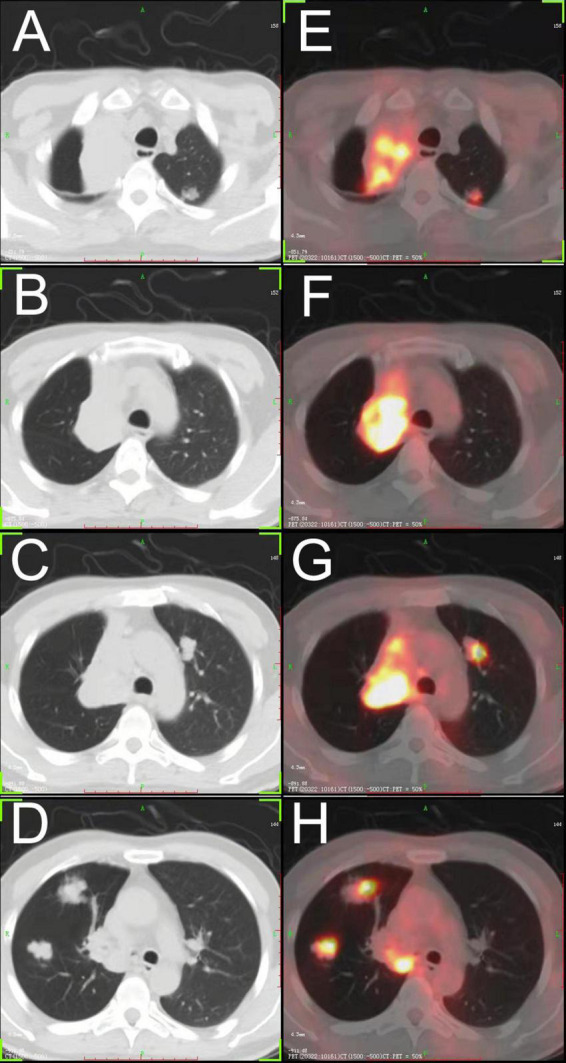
(A–D) Chest CT showing a large tumor in the hilum of the right lung, and multiple bilateral pulmonary nodules. (E–H) FDG- positron emission tomography/CT showing high accumulation of FDG in each lesion. FDG, fluorodeoxyglucose.
